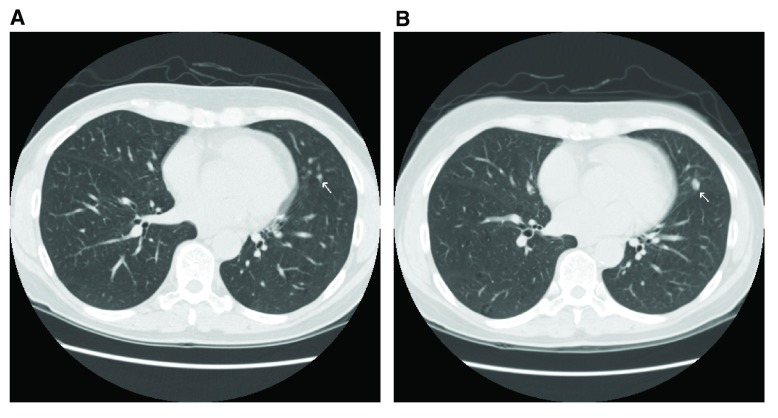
A 62-year-old woman, former smoker with a 40-pack year history, had a low-dose computed tomography (CT) screen showing a 3 mm nodule (
